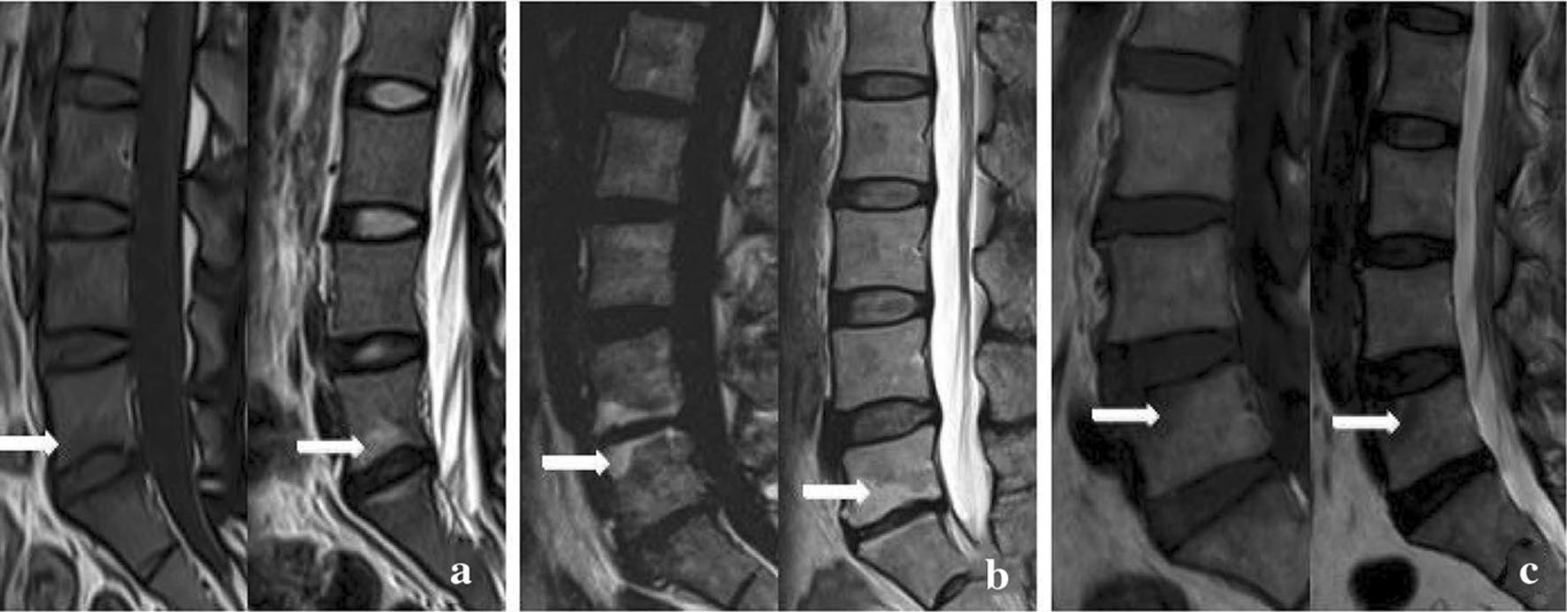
Modic change on MRI. Typical Modic changes. a Type I changes; b type II changes; c type III changes. Image courtesy of Chen Y, Bao J, Yan Q, Wu C, Yang H, Zou J. Distribution of Modic changes in patients with low back pain and its related factors. Eur J Med Res. 2019 Oct 9;24(1):34. 10.1186/s40001-019-0393-6. PMID: 31,597,571, PMCID: PMC6784341
